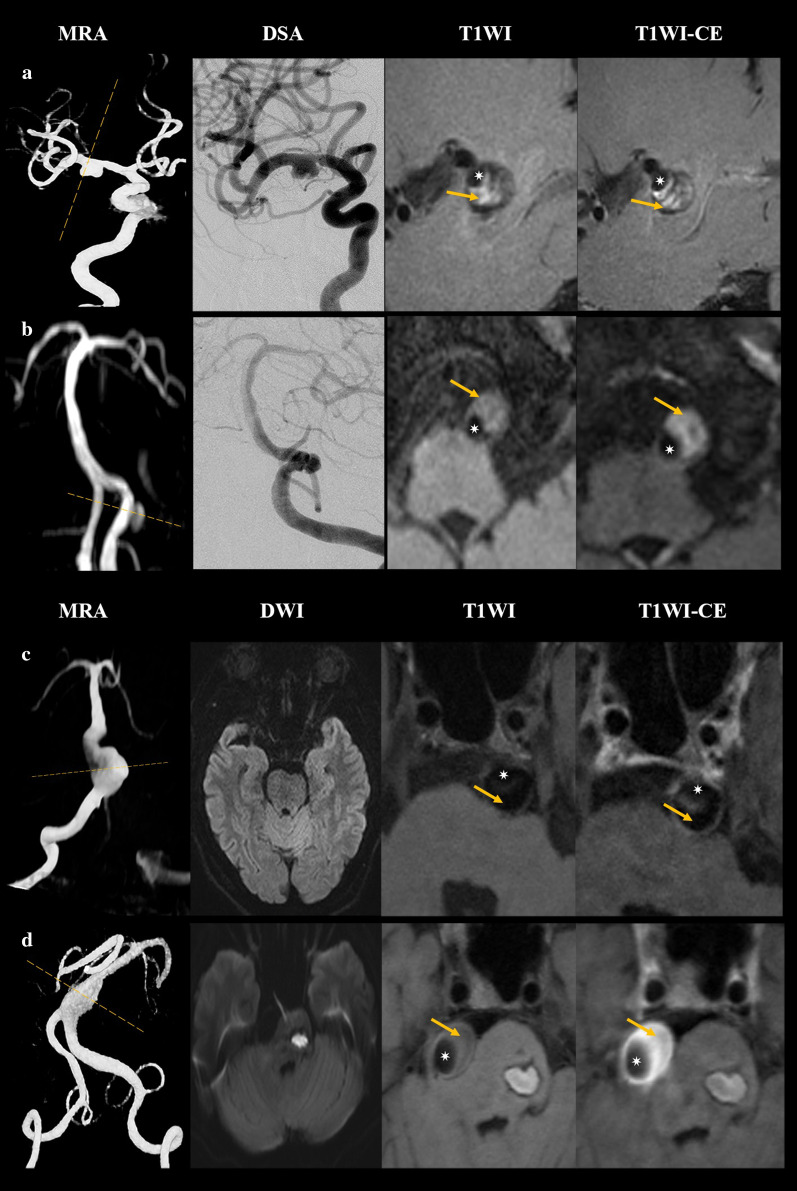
The cases of IAD. a a 51-year-old woman with acute transient ischemic attack (TIA). Both magnetic resonance angiography (MRA) and digital subtraction angiography (DSA) show an aneurysm with irregular surface on the M1 segment of right middle cerebral artery (MCA). T1-weighted and contrast enhanced (CE-T1) images show the lumen (yellow arrow) and pseudolumen (white star) on the right. b a 49-year-old man with acute ischemic symptom. Neither MRA nor DSA demonstrate an aneurysm or a dissection of the basilar artery (BA). T1-weighted and CE-T1 images show the lumen (yellow arrow) and pseudolumen (white star) on the right. c a 56-year-old man with dizziness and headache. MRA demonstrates an irregular aneurysm on the bottom of the BA, and no acute infarction was found in DWI. T1-weighted and T1-weighted enhancement (CE-T1) hrCMR are shown on the right. d a 44-year-old man with acute ischemic stroke on the left brainstem. MRA demonstrates an elliptic aneurysm on the BA, and DWI shows a sheet acute infarct in distribution of BA. T1-weighted and CE-T1 images are shown on the right
